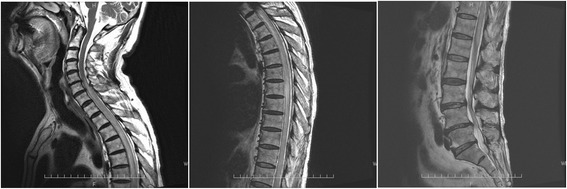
T2-weighted magnetic resonance imaging of the spinal cord showed longitudinally extensive intramedullary high-intensity areas (between C5/6 and Th12/L1)
